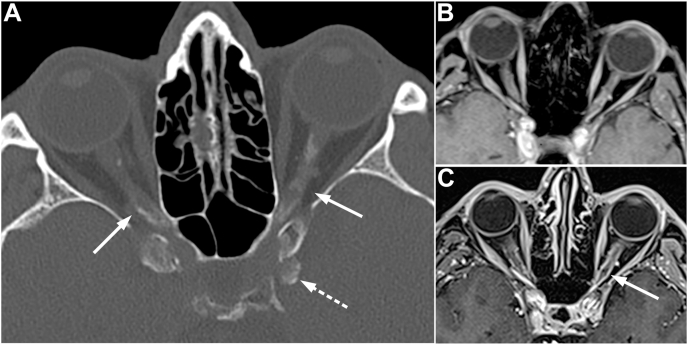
Neuroimaging of a 48-year-old man presenting with painless loss of vision in his left eye. A: Computer tomography shows calcification surrounding both optic nerves (arrows) and the left anterior clinoid process (dashed arrow). B: Initial magnetic resonance imaging (MRI) does not display contrast-enhancement or evidence of compressive lesions. C: Five years later, MRI displays progression of tram-track-like contrast enhancement surrounding the optic nerves (arrow). The final neuroradiological diagnosis was a bilateral optic nerve sheath meningioma.
